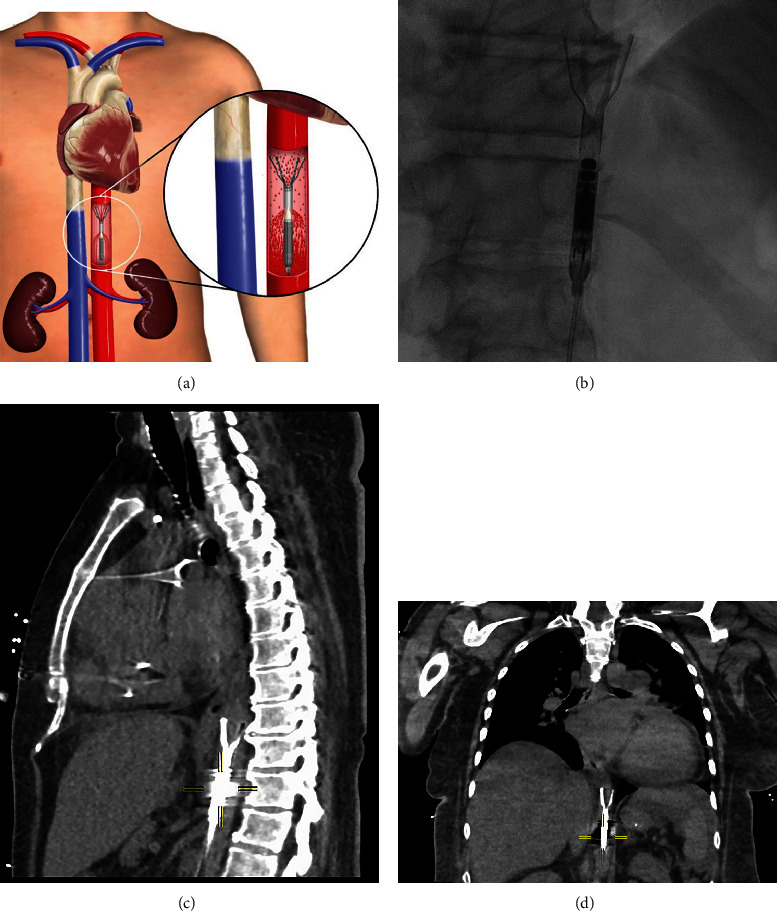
Visualization of the Aortix™ novel percutaneous mechanical circulatory support device placement in the abdominal aorta. (a) The Aortix™ device is inserted percutaneously through a common femoral artery approach and placed in the intra-abdominal aorta above the renal arteries. (b) Visualization of the device under fluoroscopy in our patient. (c) Visualization of the device (yellow marker) on a CT sagittal cross-sectional image in our patient. (d) Visualization of the device (yellow marker) on a CT coronal cross-sectional image in our patient (permission granted from Procyrion for use of copyright images). CT = computed tomography.
